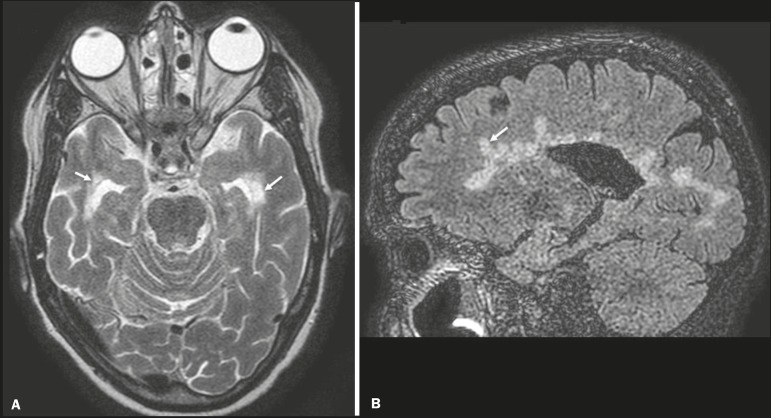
Multiple sclerosis. A: Axial T2-weighted sequence showing hyperintense signals in the periventricular and subcortical portions of the temporal lobes. B: Sagittal FLAIR sequence showing “Dawson’s fingers”.
